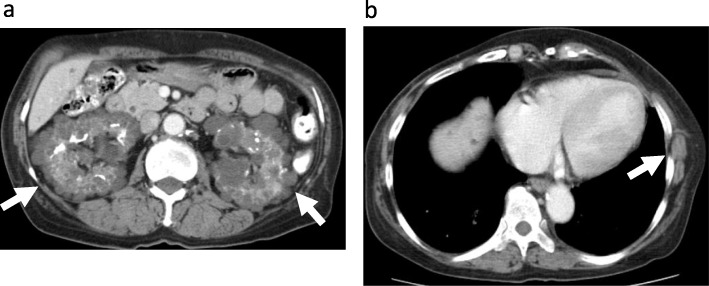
Radiologic findings in the proband. a Unenhanced computed tomography scan shows multiple kidney cysts (*arrow*) and b skin hamartoma (*arrow*)
